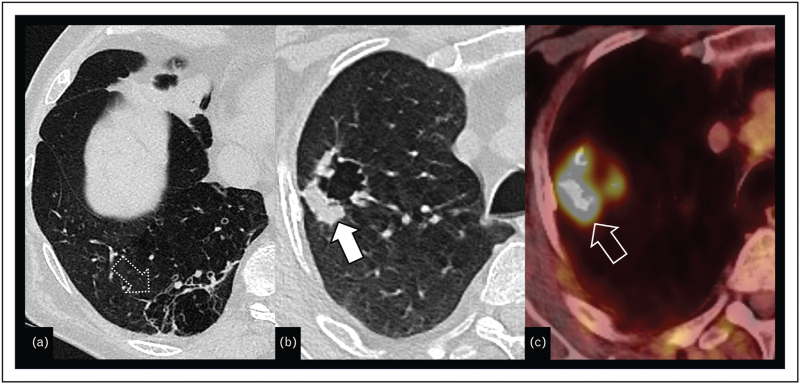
Transverse CT image reconstruction of the right lung (a) shows a thin-walled cyst (dashed arrow), without any measurable soft-tissue component. Transverse reconstruction of CT (b) and PET/CT (c) images show a cystic lesion with an asymmetric wall-thickening and a distinct soft-tissue component (white arrow), which shows an FDG-uptake (open arrow).
